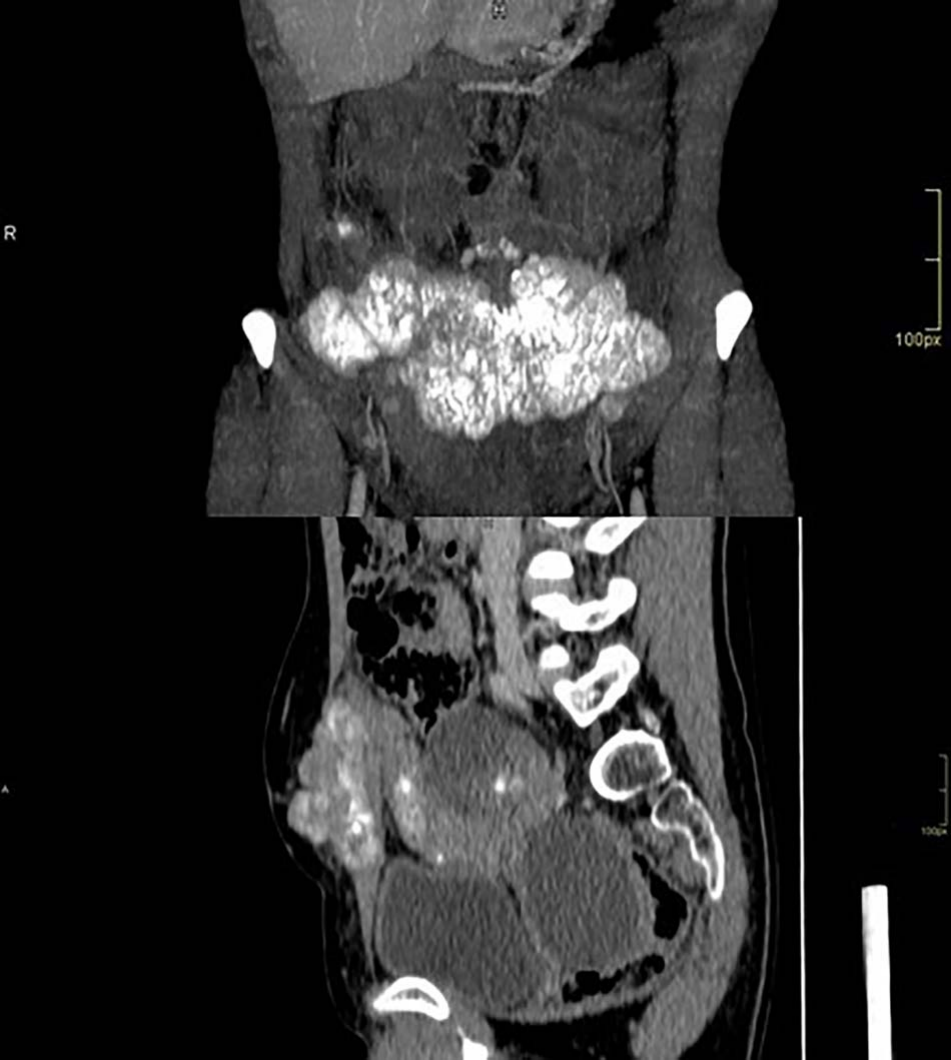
Abdominal wall CT with expansive lesion with soft tissue density, heterogeneous, with the appearance of a cluster of nodules, measuring 16.4 × 5.1 × 7.7 cm (334 cm^3^ volume) in the major latero-lateral, antero-posterior axes and longitudinal, respectively.
